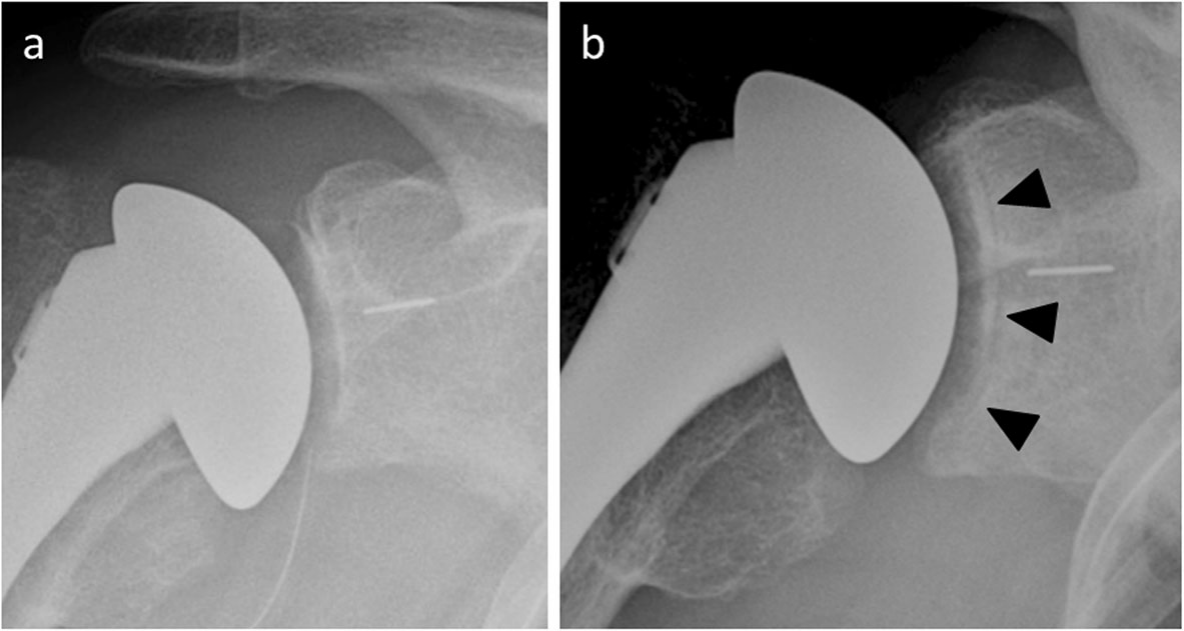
a Normal postoperative anteroposterior shoulder radiograph of a 51-year-old man with total shoulder arthroplasty. b Anteroposterior radiograph in the same patient as a 4 years later shows a radiolucent line around the glenoid implant (arrowheads), while the patient is asymptomatic
